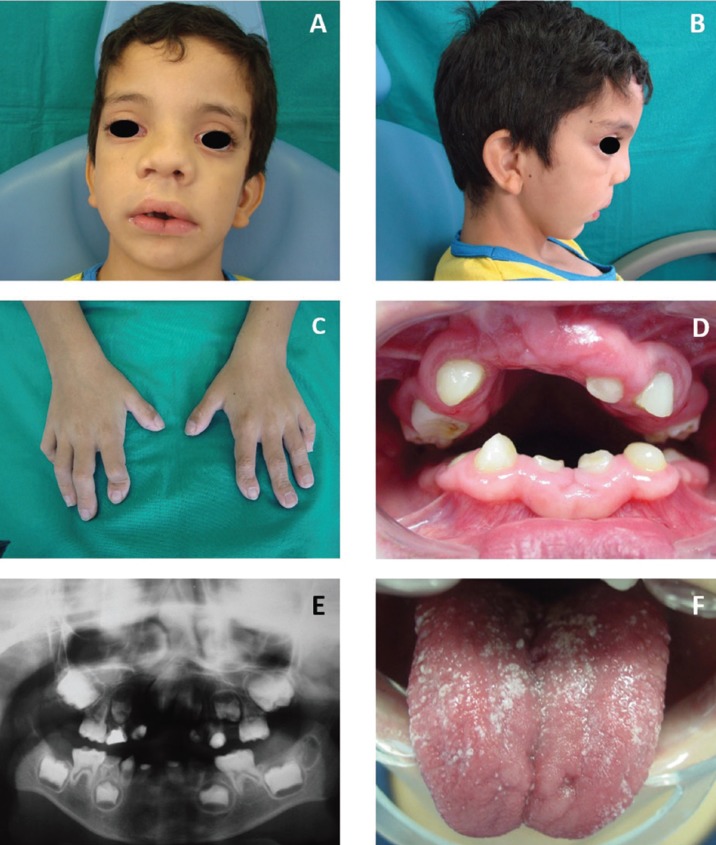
Patient #5. A) Frontal view of the face showing long palpebral fissure with eversion of the lateral third of the lower eyelids, hypertelorism, strabismus, sparse eyebrows in the lateral third, wide nose and surgical scar of a cleft lip-palate correction. B) Lateral view of the face showing protruding ears with low implantation and concave profile. C) View of the patient’s hands showing brachydactyly of the fifth finger. D) Intra-oral view showing spaced teeth, absence of teeth and enamel hypoplasia. E) Panoramic radiograph showing dental agenesis. F) Bifid tongue with pseudomembranous candidiasis.
